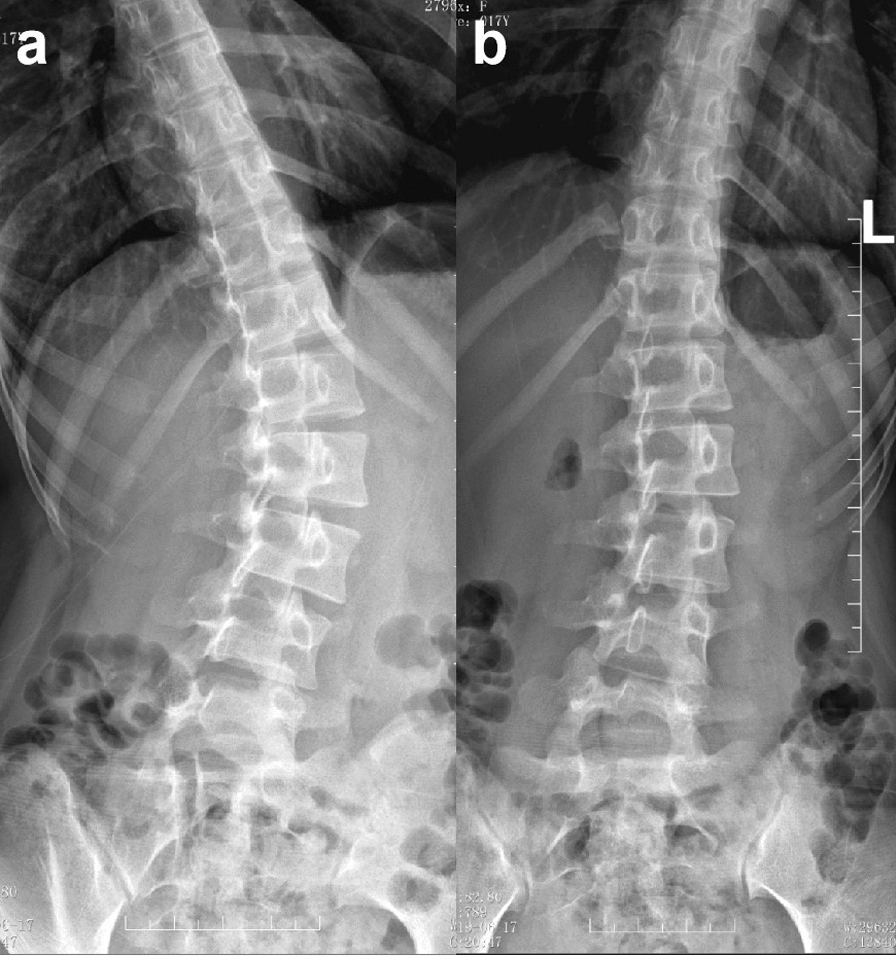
The right lateral bending X-ray showed that the lumbosacral curvature was 15.1° (a), and the left lateral bending X-ray showed that the lumbar curvature was 4.6° (b). The flexibility of the main lumbar curvature was 85.7%
